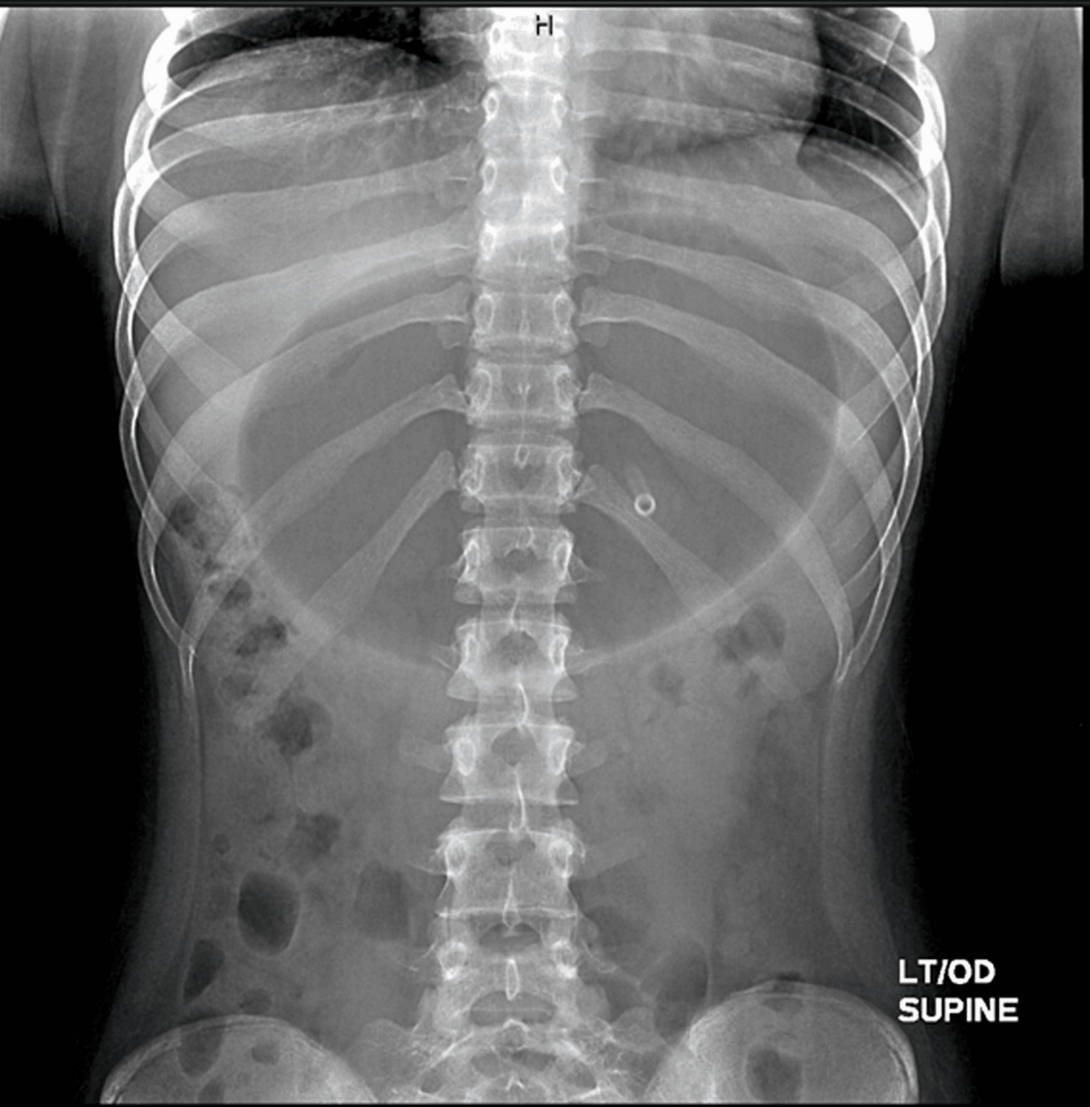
Anterior-posterior x-ray of the intragastric balloon
